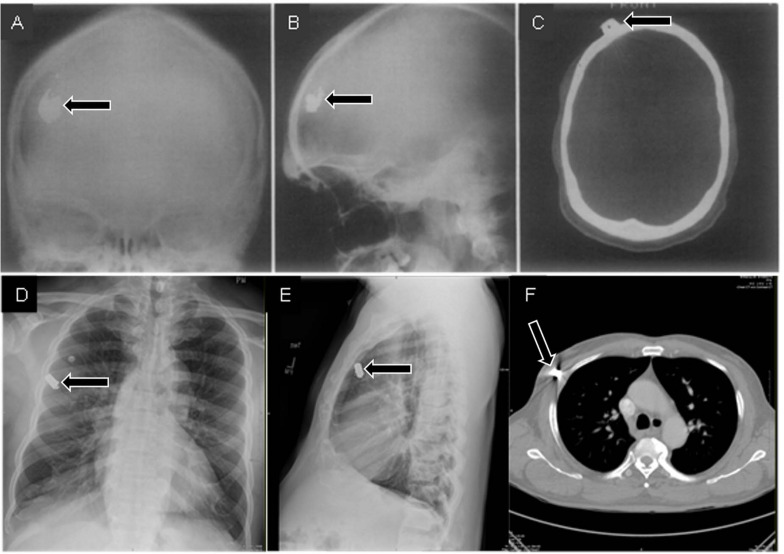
Frontal (A) and lateral (B) radiographs of a patient who suffered a gunshot wound to the skull seem to indicate that the bullet is within the skull. However, the skull does not appear to be fractured, and there is no indication that the bullet actually passed through the skull. A CT scan (C) provided the critical viewpoint to conclude that the bullet was just underneath the scalp (A–C from Daffner, 1989). In a separate case, frontal (D) and lateral (E) radiographs seem to indicate that a bullet is within the lung. However, a CT scan (F) revealed that the bullet was in the surrounding tissues.
